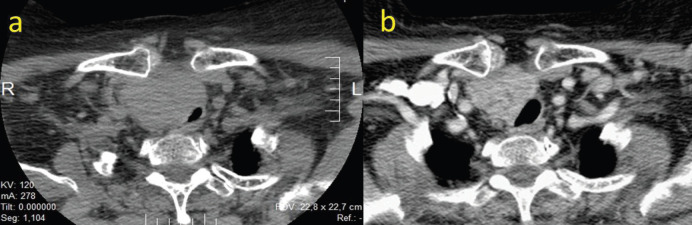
(a): CT scan of the neck pre-neoadjuvant therapy. (b): CT scan after 10 weeks of lenvatinib treatment (24 mg/day).
